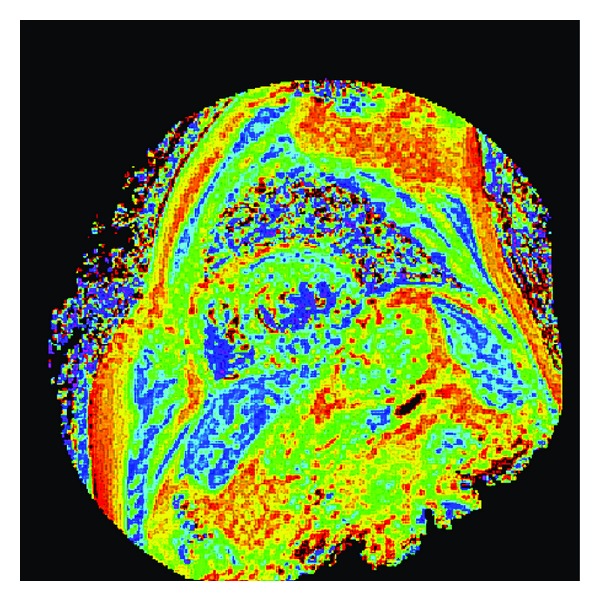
Myocardial T2 mapping generated from multiecho T2-weighted imaging.
